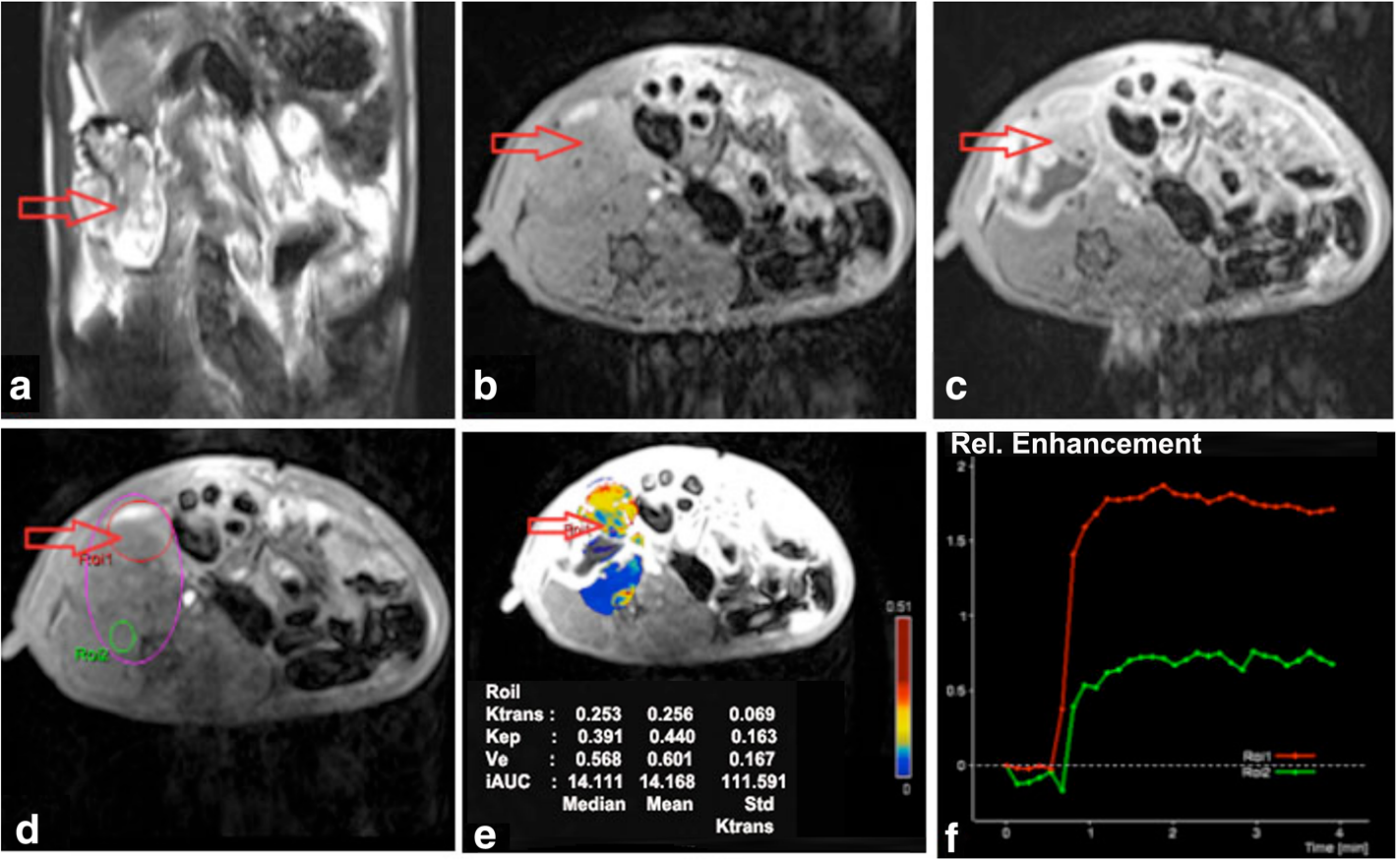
DCE-MRI imaging of EOCs with TIC III. In the right adnexal area, a multilocular cystic solid was observed on T2WI with fat suppression (a) and T1WI (b) on the coronal position, showing obvious enhancement and a solid portion on contrast-enhanced T1WI with fat suppression (c). In DCE-MRI images, ROI, including ROI1 (ovarian lesion) and ROI2 (muscle), were selected (d); pseudo-color images can be obtained by quantitatively analyzing DCE-MRI (e); the dynamic curve appeared as an initial steeper increase followed by a plateau (f). The red arrow points to the tumor focus
